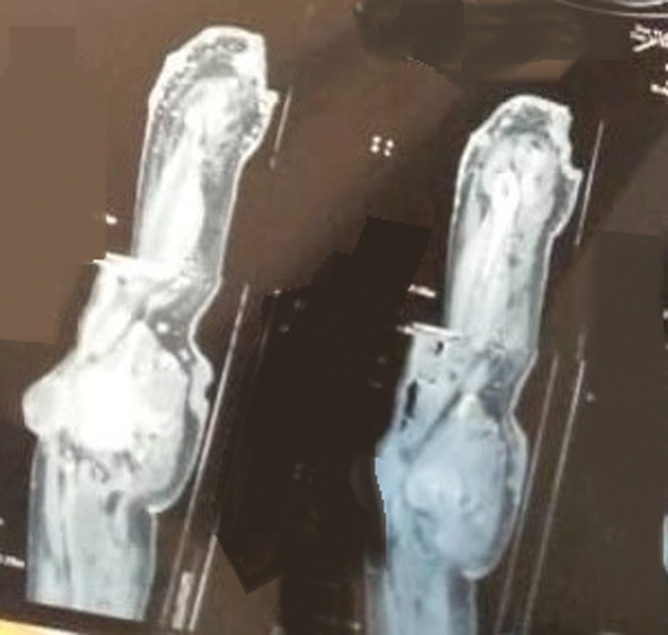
pelvic stage of a CT scan, axial section, showing the buttock infiltrating the soft tissues of the gluteal region, inguinal adenopathies
